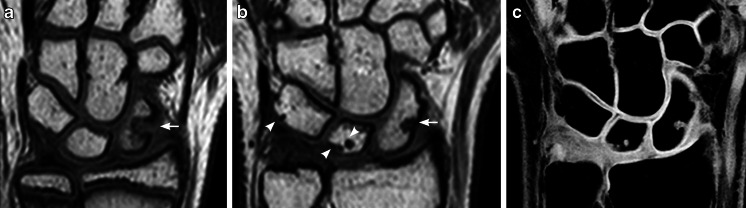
Proximal carpal row. a, b Coronal T1-weighted turbo spin-echo MR image in a 13-year-old boy (a) shows a large bony depression in the scaphoid bone (*arrow*). A 4-year follow-up (b) the depression is still seen but is less pronounced. New bony depressions are seen on the surface of the lunate and triquetral bones (*arrowheads*). c Coronal water-selective (coronal volumetric steady-state spoiled gradient echo) cartilage sequence shows that both old and new depressions are covered with cartilage
